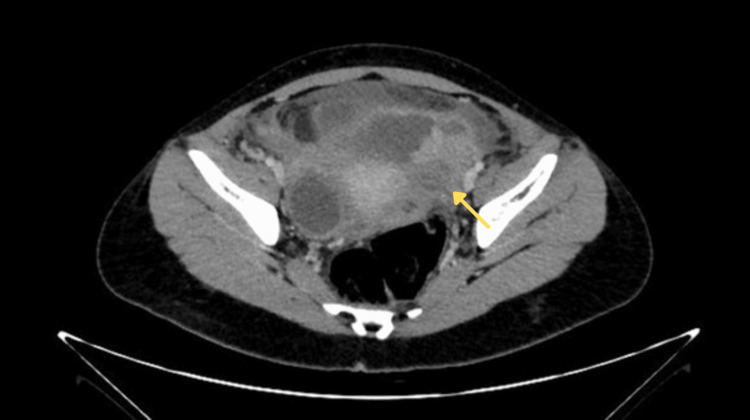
Contrast-enhanced axial computed tomography (CT) scan of the abdomen and pelvis demonstrating a heterogeneous left adnexal mass (arrow) measuring approximately 46 × 38 × 30 mm, associated with peritoneal thickening and ascites.
